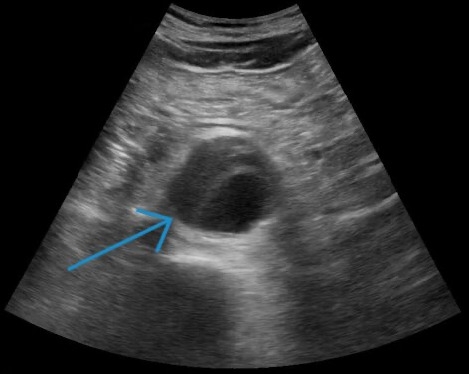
Point of care ultrasound (POCUS) demonstrating a 5.0 x 5.3 cm abdominal aortic aneurysm.
